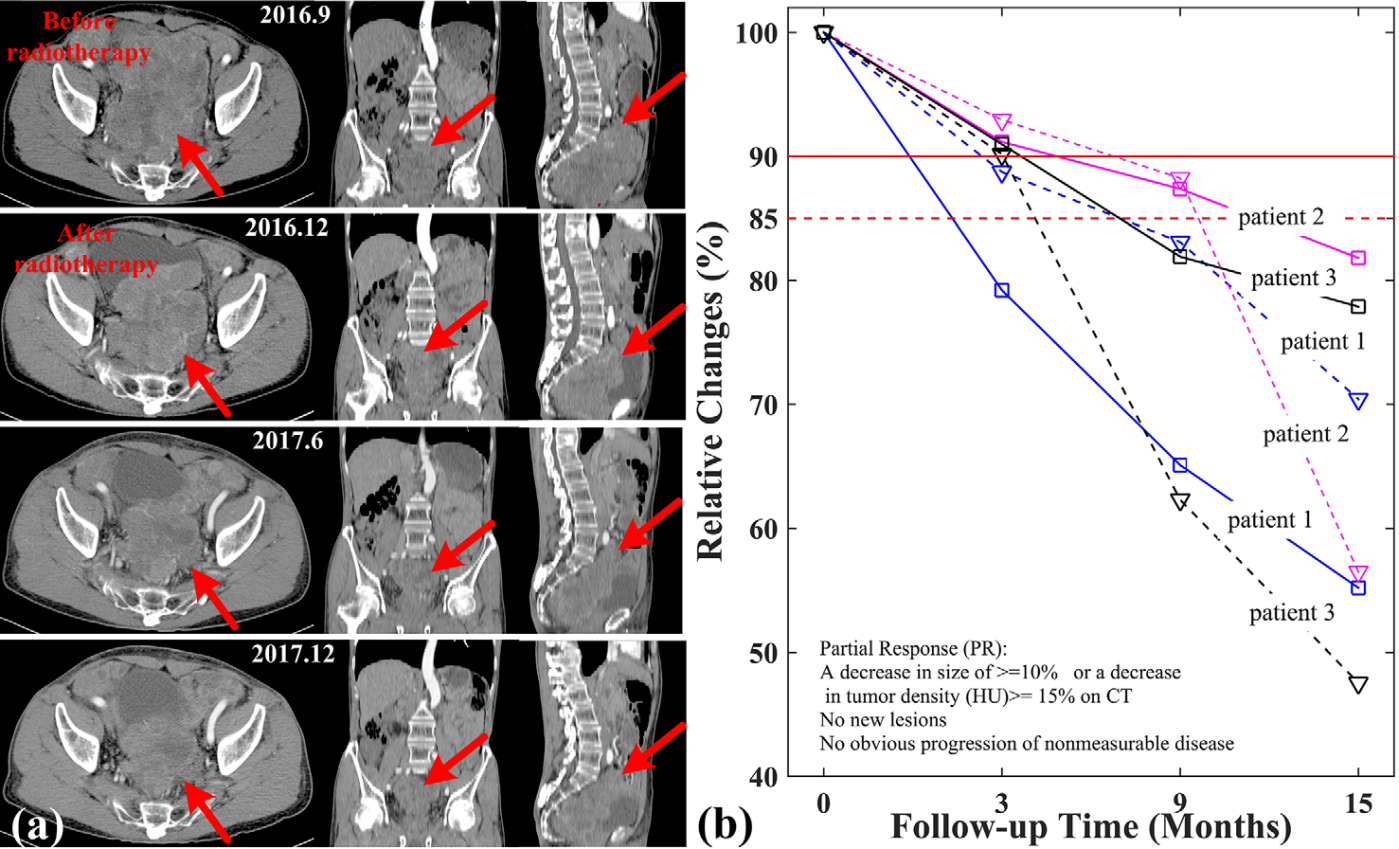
Relative changes of tumor size and CT values in three patients. (A) CT imaging of patient 1; (B) Relative changes of tumor size (solid line) and CT (dashed line) values in three patients.
